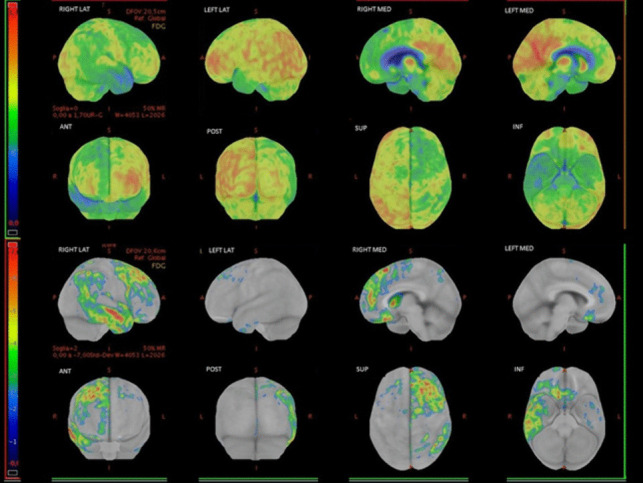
Brain FDG-PET of case II-8, showing right fronto-temporal involvement. Images have been obtained with CortexID suite (GE Healthcare). Hypometabolic areas appear in cold tones in the upper rows, representing FDG uptake. The lower rows represent the z-score map obtained by comparison with the software database of normal subjects: the higher the z-score (red), the more pronounced the reduction of the metabolism
